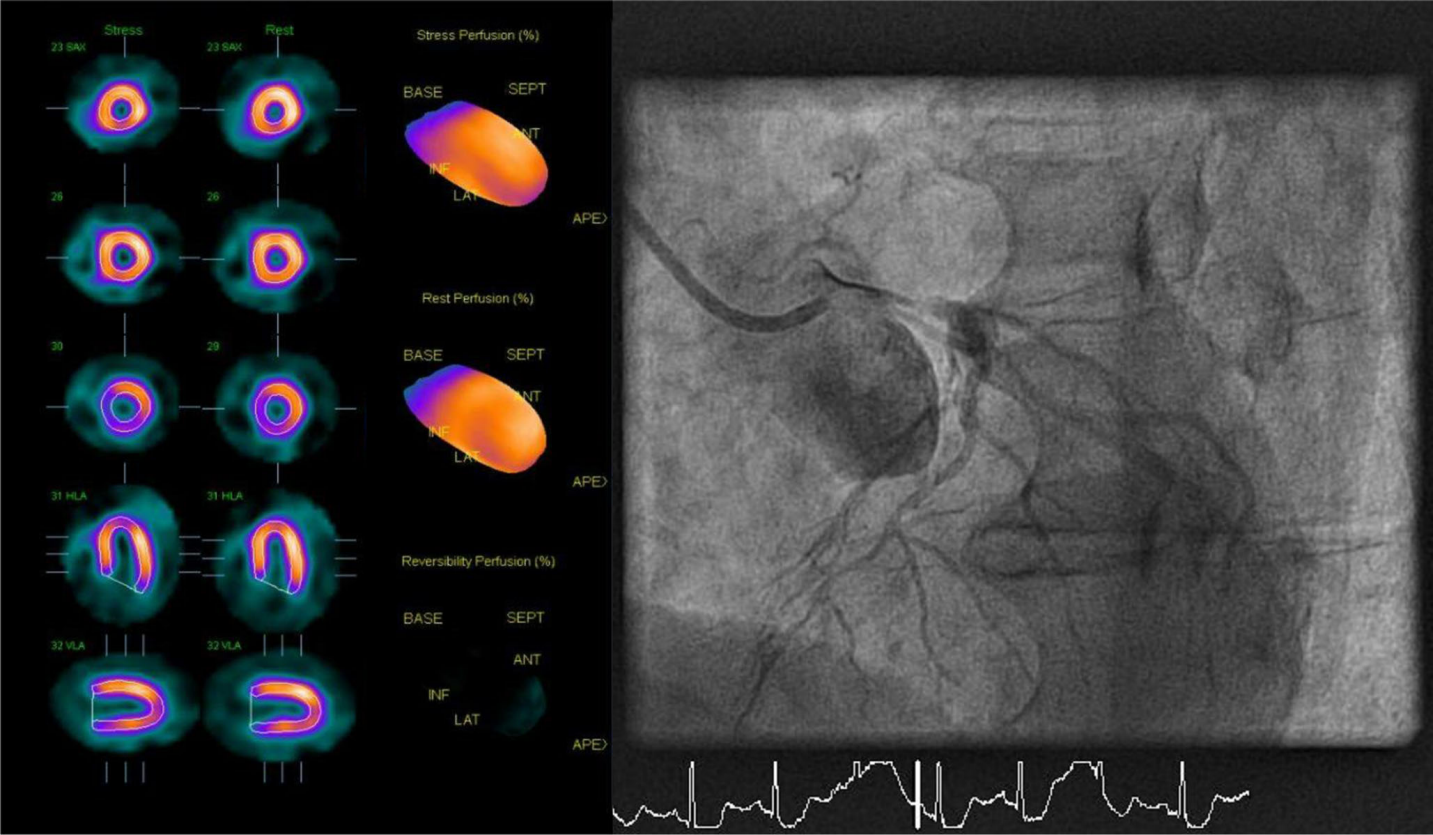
A 73-year-old male with atypical angina without cardiovascular risk factors and no prior cardiac history was evaluated for coronary artery disease with ^99m^Tc-sestamibi single photon emission computed tomography (SPECT) during rest and vasodilator stress (adenosine). Tracer distribution was homogenous during rest and stress, and the visual and automated grading of the images yielded a normal test result. Given the intermediate-to-high pre-test likelihood of disease and persistent symptoms, the patient was referred for invasive coronary angiography. Upon engagement of the left main coronary artery, the catheter wedged the artery and the patient became ischaemic and hypotensive. Subselective angiography displayed a subtotal occlusion of the left main. SPECT imaging was false-negative due to balanced ischaemia
